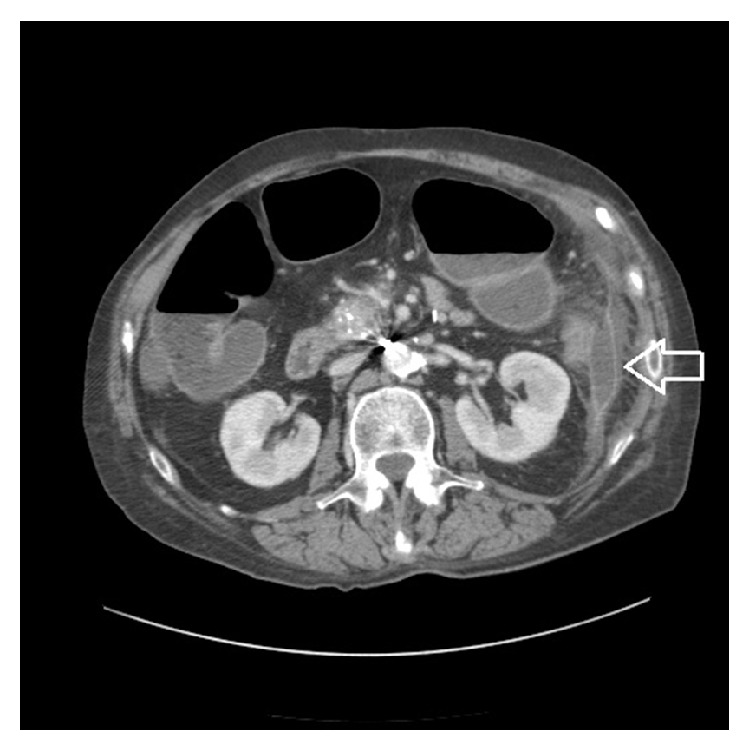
CT abdomen/pelvis done at outside hospital at time of admission showing fluid collection around the pancreatic tail and splenic flexure 4.7 cm.
